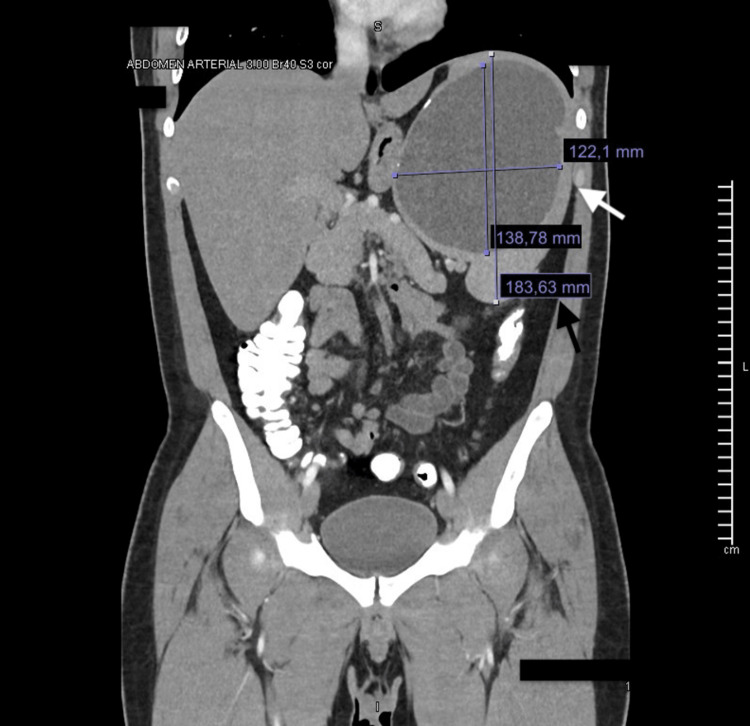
Contrast-enhanced computed tomography (coronal view). Splenic measurement of 183.63 mm (black arrow). Splenic cyst measurement of 122.1 × 138.78 mm (white arrow).
